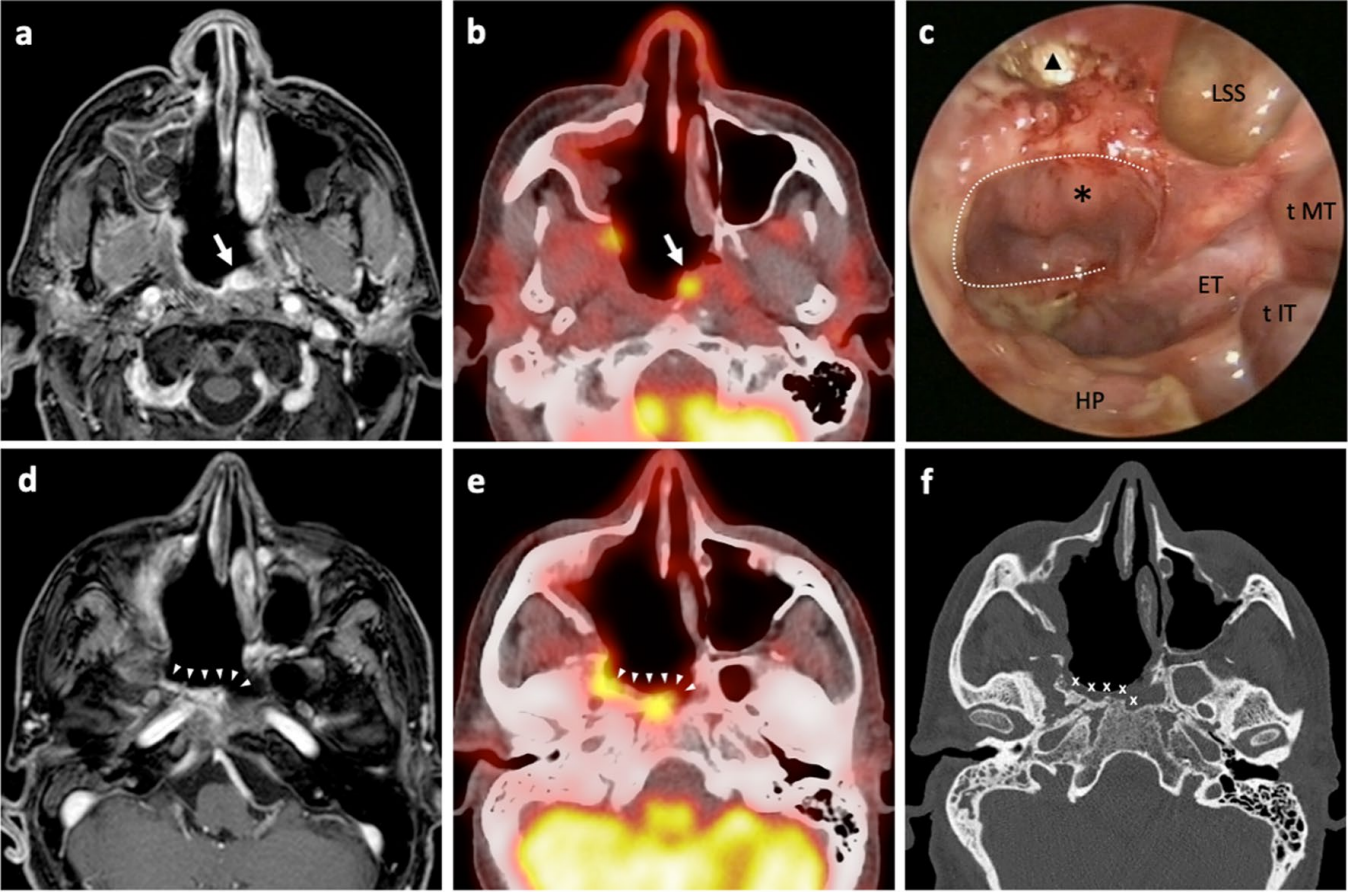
Radiological and clinical follow-up performed nine months after NER type 3 (right side) and ipsilateral MRND for recurrent uNK-NPC (rpT1N3cM0) in a 48 year old patient. An area of focal contrast-enhancement (a) and ^18^FDG uptake (b) was evident at the level of the left portion of the nasopharynx (white arrow), corresponding at the endoscopic evaluation (c) to a small swelling (black asterisk) below the left pedicled nasoseptal flap (white dotted line). Imaging investigations showed another area of diffuse contrast-enhancement (d) and ^18^FDG uptake (e) at the level of the right pterygoid area (white arrowheads) which, at the endoscopic evaluation (c), appears as an area of exposed necrotic bone and granulations (black triangle). Biopsies were taken from both areas of suspect signal, resulting in local recurrence of uNK-NPC at the level of the left nasopharynx and chronic inflammation at the level of right pterygoid, due to underlying osteonecrosis (white crosses) as demonstrated by CT scan (f). *ET* Eustachian tube, *HP* hard palate, *LSS* left sphenoid sinus, *tIT* tail of inferior turbinate, *tMT* tail of middle turbinate
